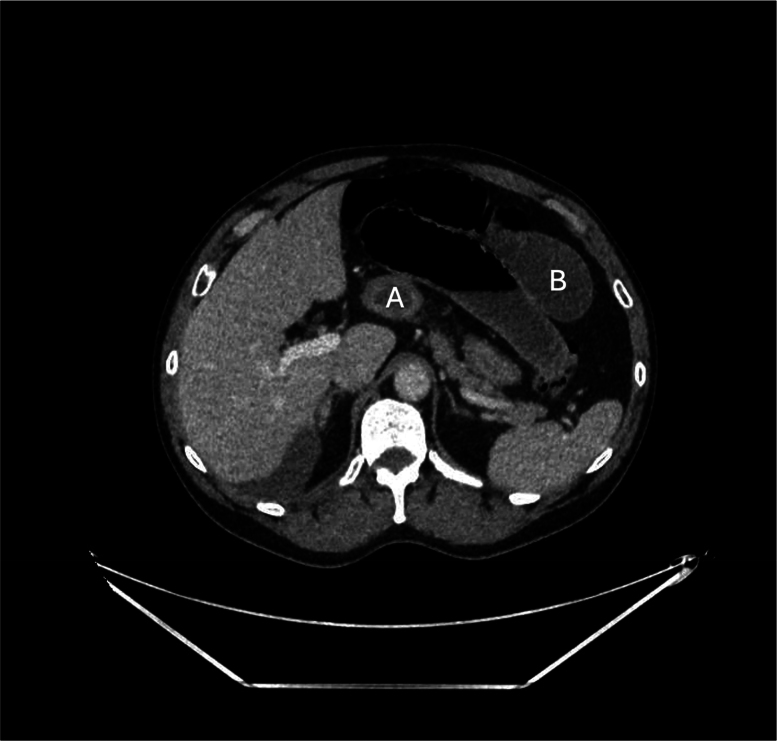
Axial section of computed tomography scan showing terminal ileum (A) herniation through the epiploic foramen (foramen of Winslow), with dilated small bowel loops (B).
